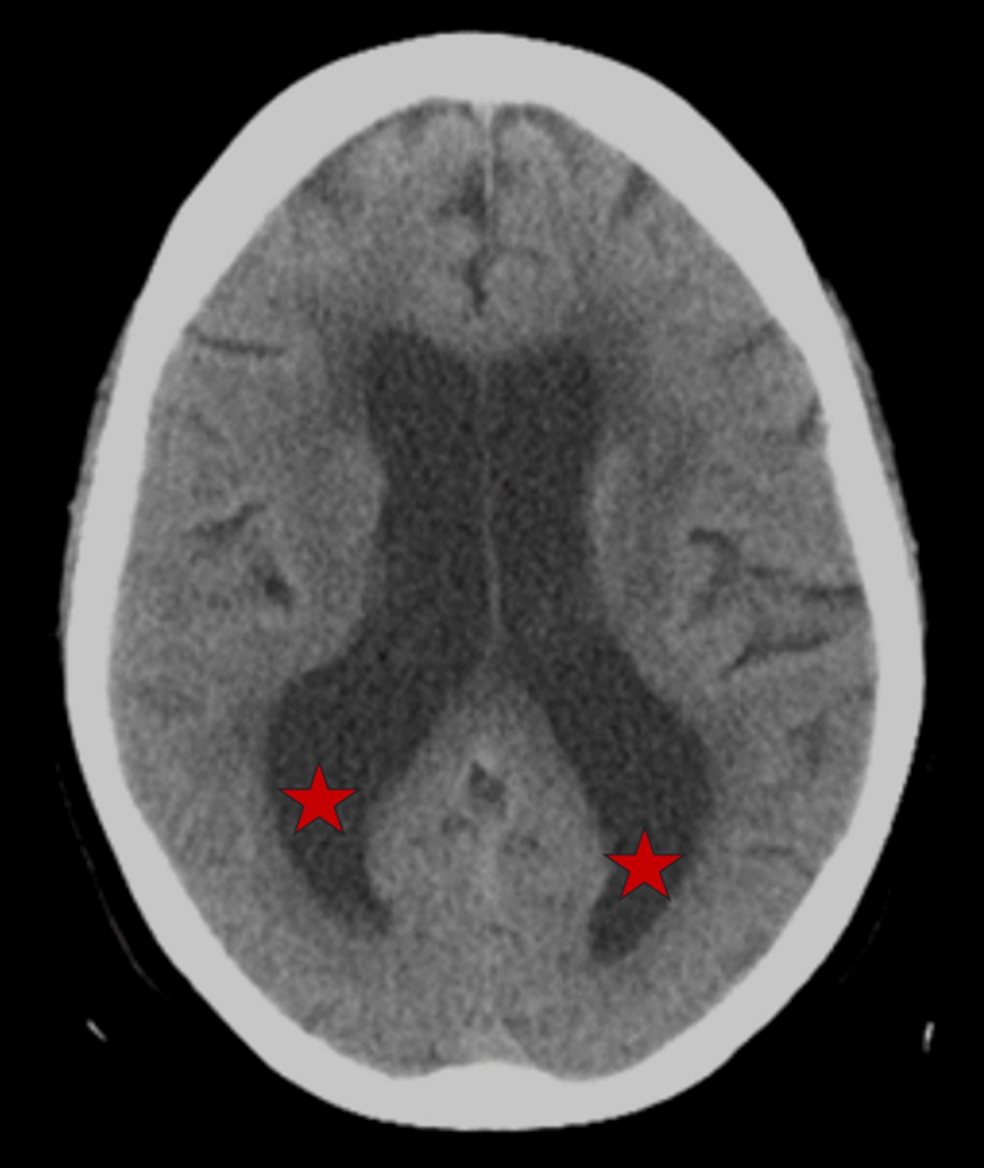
CT scan demonstrating enlargement of the lateral ventricles, particularly in the posterior horns (red stars) in the patient with normal pressure hydrocephalus.
